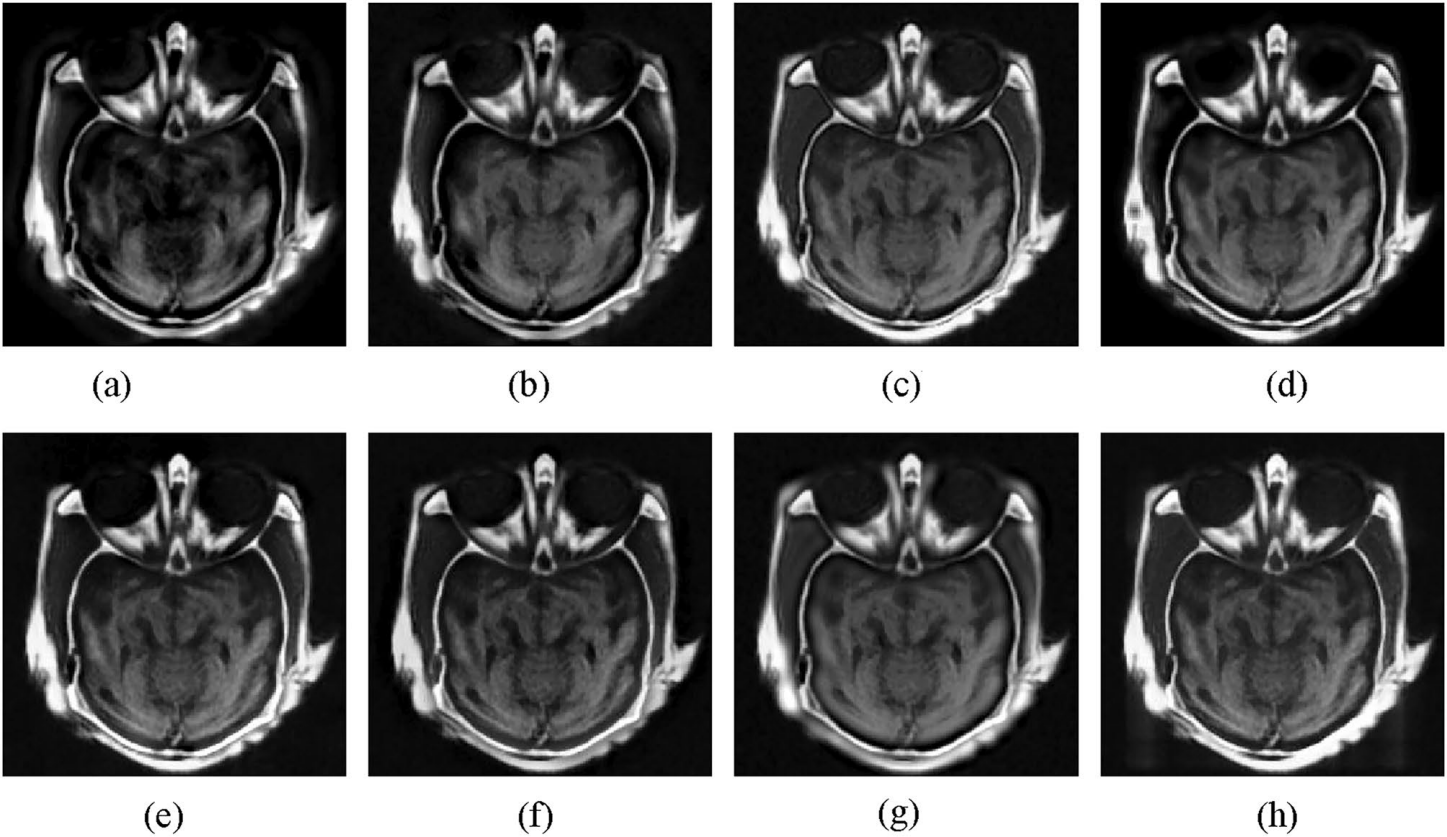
Fusion results of different methods for CT and MRI images. (a) CNN-NSCT. (b) NSST. (c) NSST-ST. (d) SR. (e) MST-SR. (f) GFF. (g) DSWT-RBFN. (h) Our method.
